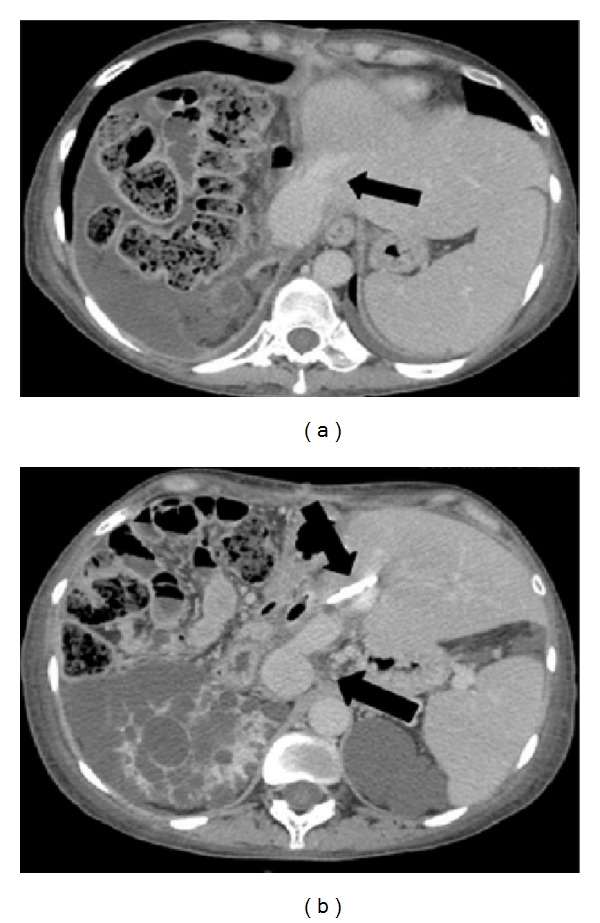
CT scan of combined left kidney-left liver transplantation: arrow of Figure 3(a) shows the left hepatic vein; arrows of Figure 3(b) show the biliary stent and the portocaval shunt.
